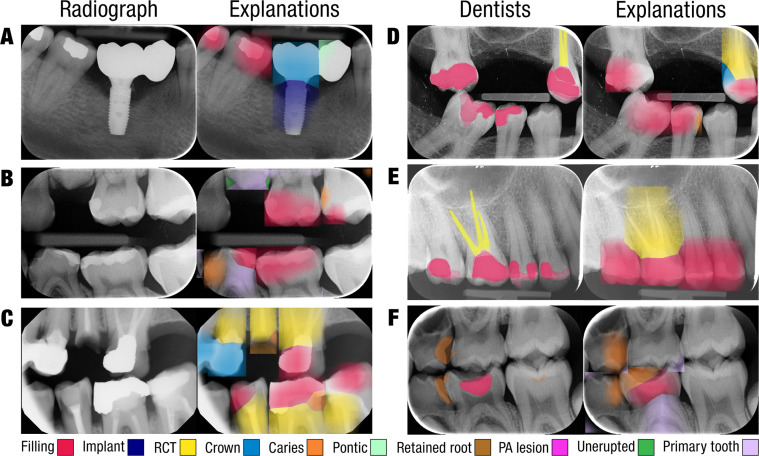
(A-C) Grad-CAM explanations of dental findings in held-out intraoral radiographs. Predicted teeth in each intraoral radiograph were processed by the dental finding classifiers to determine the presence or absence of ten dental findings. For each present finding, the corresponding Grad-CAM was superimposed on the radiograph with a finding-specific color, see the legend at the bottom. To improve readability, each Grad-CAM was limited to the tooth’s predicted bounding box and colors were not mixed. (D-F) Comparison of dentist segmentations and Grad-CAM explanations for held-out intraoral radiographs. Three dentists segmented fillings, periapical (PA) lesions, root canal treatments (RCTs), and caries lesions, which are visualized with color maps where the intensity of the color represents the number of dentists that segmented the pixel.
